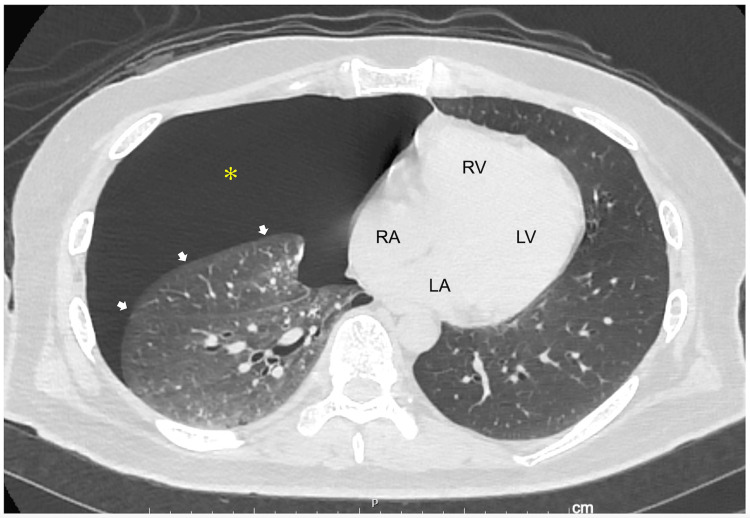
An axial computed tomography image of the chest on admission showing right pneumothorax A right pneumothorax (asterisk) with the build-up of air between the collapsed right lung (arrows) and chest wall is shown. The heart was suspected to develop a leftward shift. LA: left atrium; LV: left ventricle; RA: right atrium; RV: right ventricle
